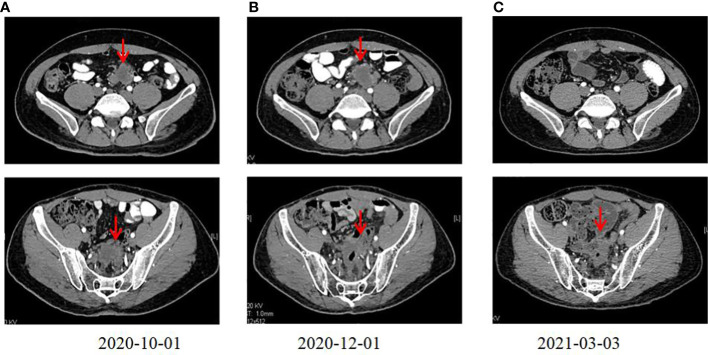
CT scanning showing the chronological response of the patient after a cycle of treatments. (A) CT before radiation. (B) CT after ten days of radiation revealing lesions. (C) CT revealing an abscopal effect following the sequential immunotherapy performed.
